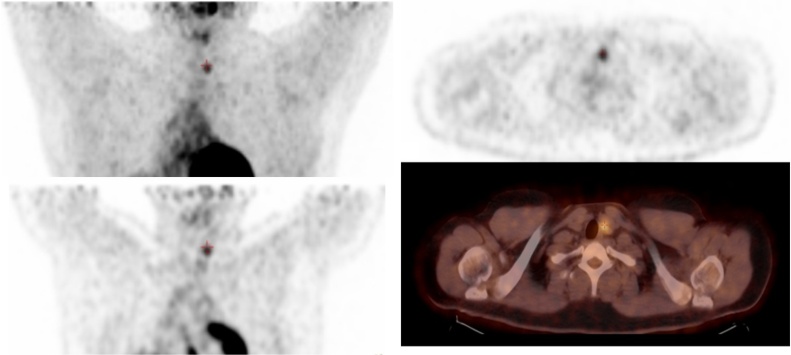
20 years old male, a case of atypical carcinoid, status post-surgical resection of right middle and lower lung lobes. F-18 FDG PET/CT showed a hypermetabolic left thyroid lobe nodule with SUVmax of 4.2 and CT HU of 32. FNA cytology revealed a benign follicular nodule.
